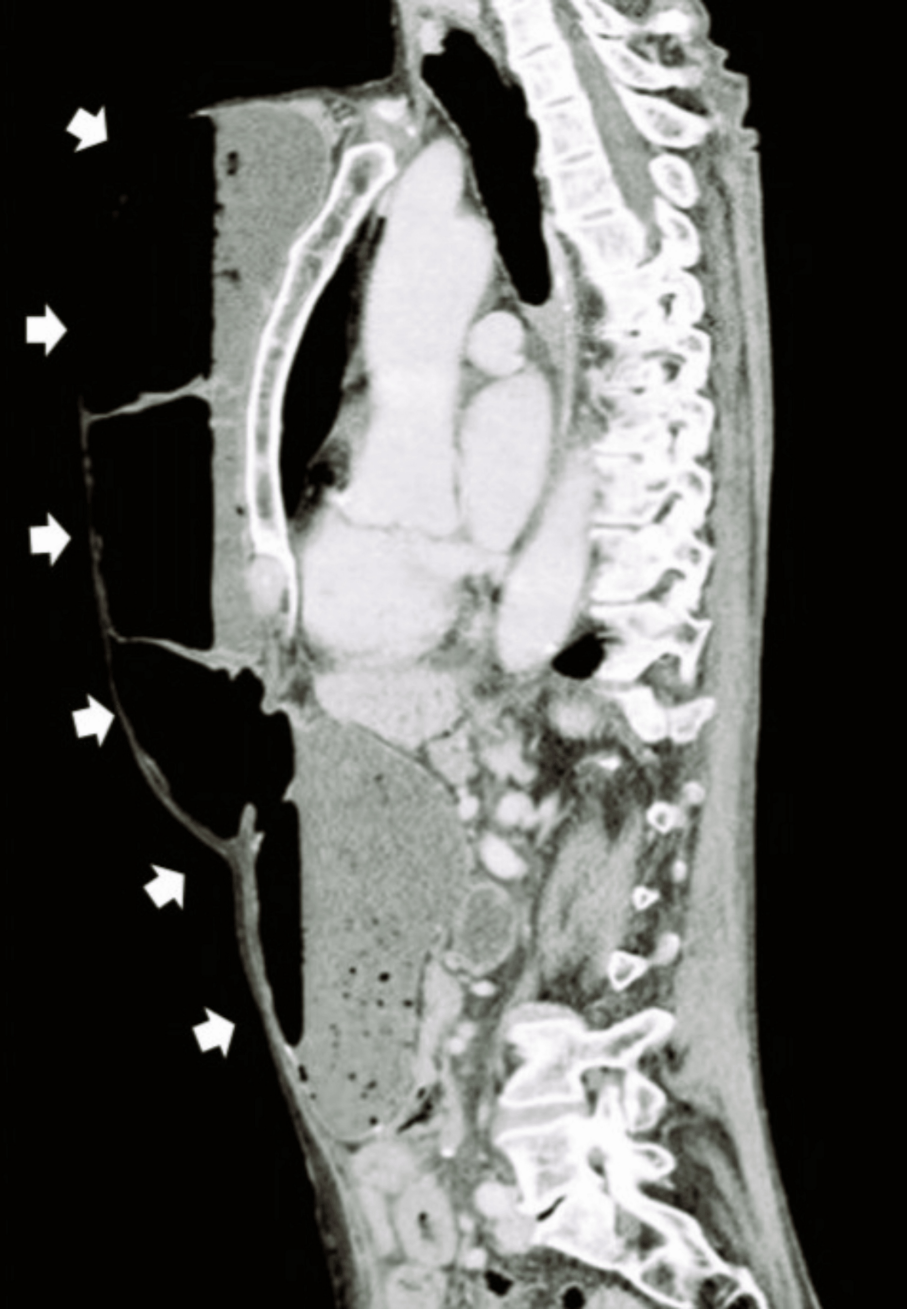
Sagittal Thoracoabdominal CT - Marked Neoesophageal Distension Contrast-enhanced thoracoabdominal CT showing significant distension of the interposed colonic conduit with no signs of perforation or ischemia, suggestive of mechanical obstruction, as indicated by the white arrows.
